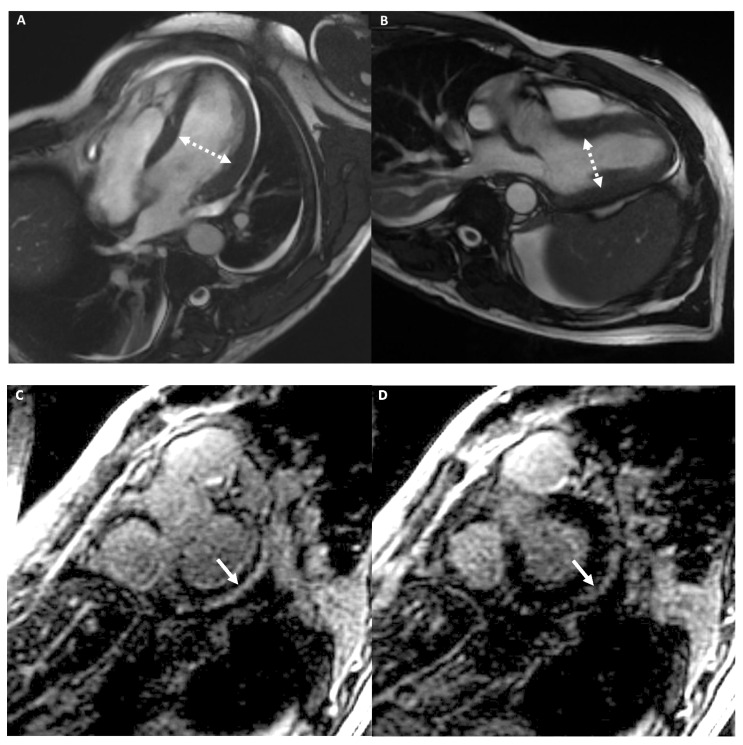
A 39-year-old male athlete (cyclist) with palpitations. Cine-CMR images show mild LV dilation with increased dimension in four-chambers (A) and in LV outflow-tract (B) plane (dashed double-headed arrows). Delay-MRI contrast images demonstrate, in short-axis planes (C,D), a subepicardial linear LGE at the basal infero-lateral LV wall (arrow). The final diagnosis was LDAC.
